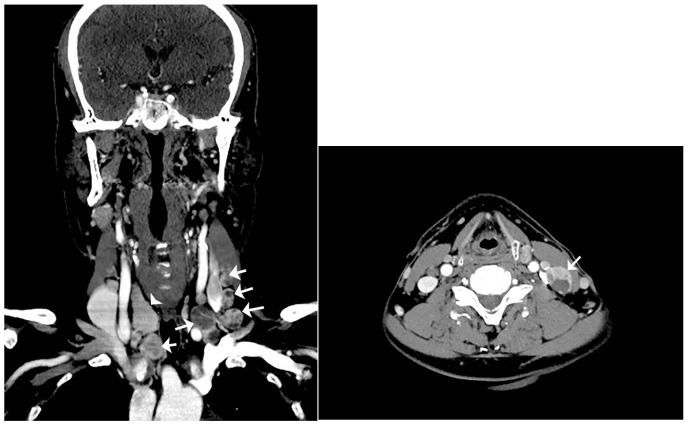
Computerized tomography (CT) scan of the neck. Left: coronal CT scan of the neck reveals multiple enlarged lymph nodes with internal variable cystic change involving bilateral neck of right level III and left levels II, III, and IV, and right lower cervical chain (arrow: multiple enlarged lymph nodes with cystic change; arrowhead: right lobe of thyroid). Right: axial CT scan of the neck reveals an enlarged lymph node with internal cystic change at left level III (arrow: the most enlarged lymph node with cystic change). Therefore, total thyroidectomy and cervical lymph node dissection were performed to distin-guish lymph node metastasis of occult thyroid cancer from seeding of thyroid tissue due to pre-vious thyroid surgery. Resected bilateral cervical lymph nodes were enlarged to the maximum dimensions of 3.5 × 1.7 × 1.0 cm. The cut surface of the lymph node and thyroid gland showed multiple variable-sized pale tan to brown solid nodules with cystic changes (Figure 3).
